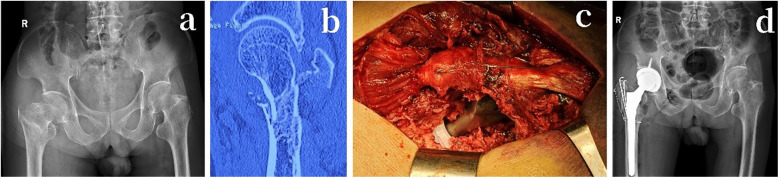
An 78-year-old male patient with a right-sided Evans-Jensen type IV intertrochanteric femur fracture due to a fall. (a) Preoperative anteroposterior x-ray of both hip joints. (b) Preoperative coronal CT scan. (c) Intraoperative total hip arthroplasty, with the use of Kirschner wires for tension band fixation of the greater trochanter and titanium cables for securing the proximal femur. (d) Postoperative anteroposterior x-ray of both hip joints on the first day after surgery.
